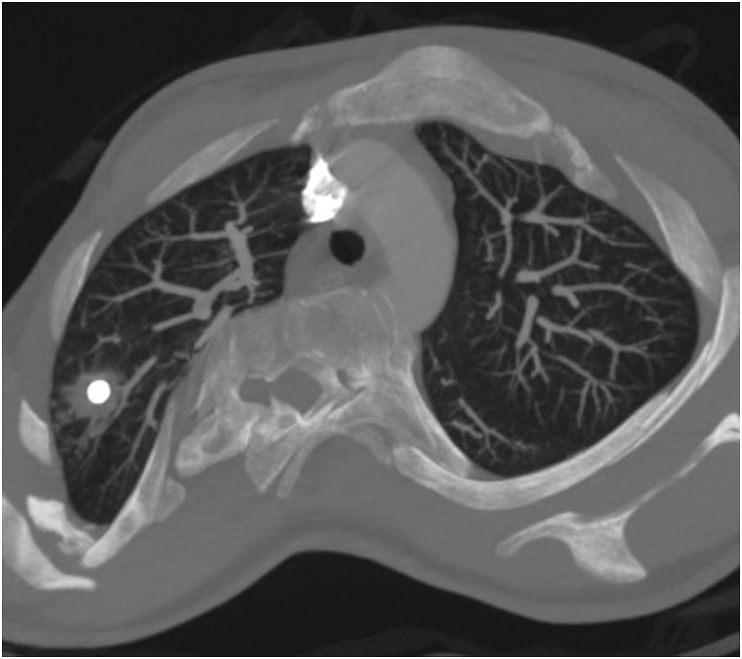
Axial maximum intensity projection (MIP) CT image shows the upper edge of spinal rod within the right upper lobe surrounded by consolidations corresponding to injured parenchyma.
